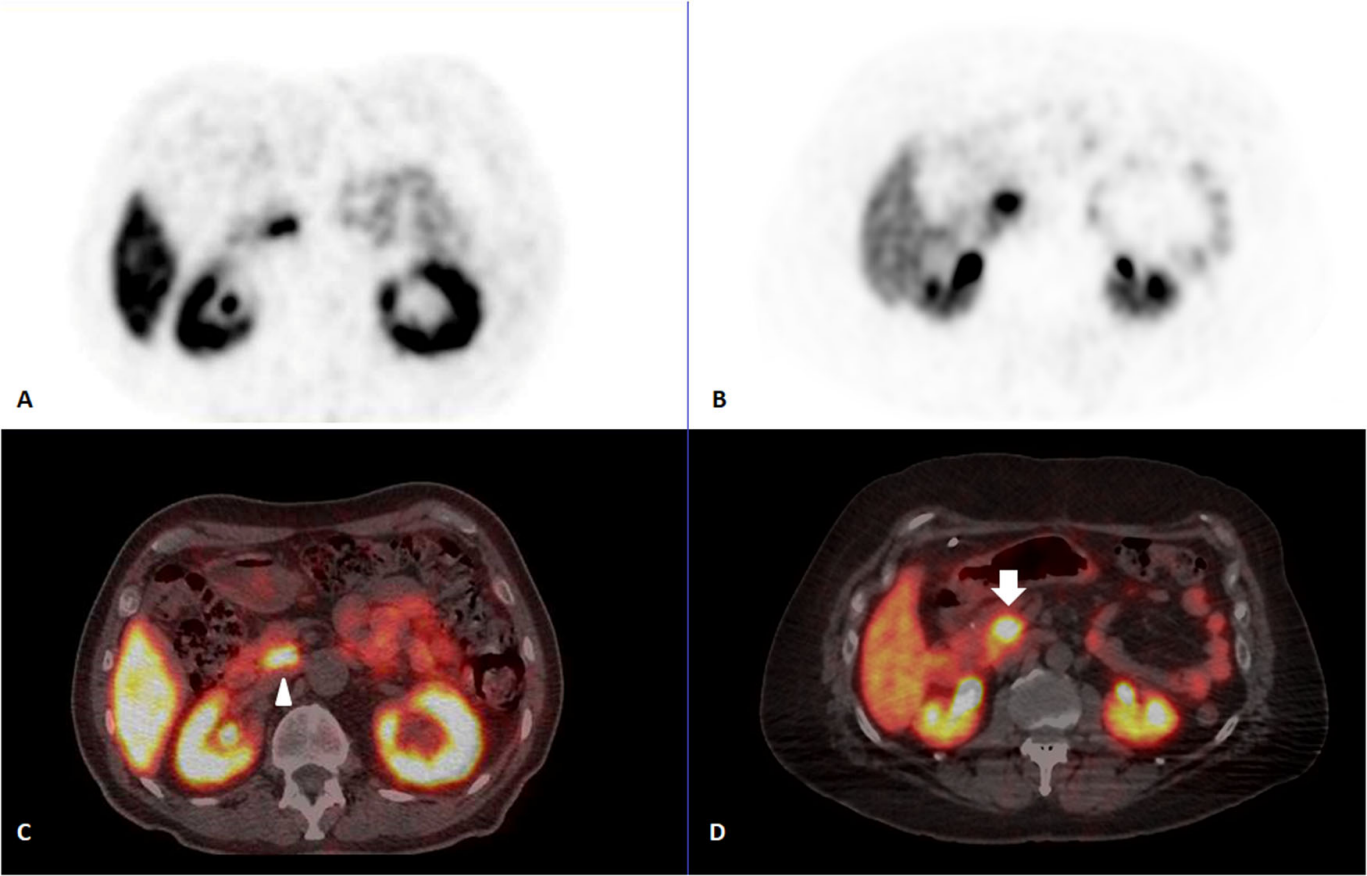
Example of physiological uptake of the uncinate process of the pancreas on 68Ga-DOTATOC PET/CT (arrowhead, PET alone–image A, fused PET/CT–image C) and tumoral uptake of the uncinate process on 68Ga-DOTATOC PET/CT (plain arrow, PET alone–image B, fused PET/CT–image D)
